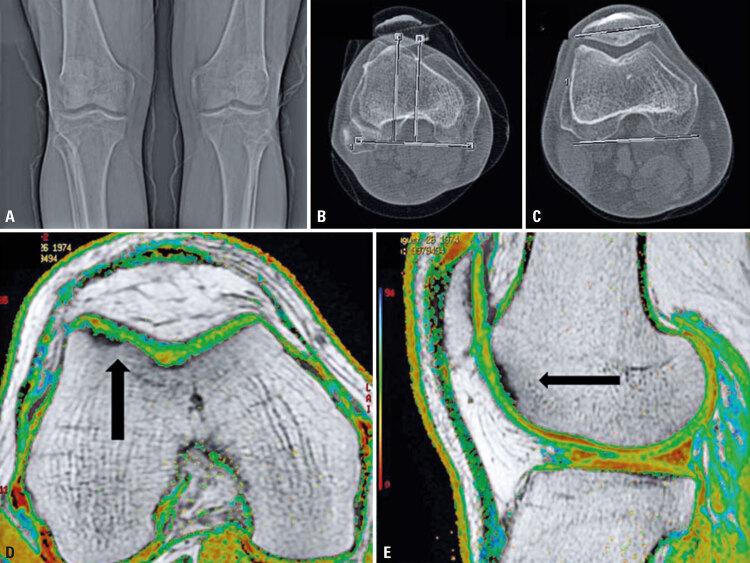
Right knee images before surgery. (A) X-ray with no sign of osteoarthritis; (B) Computed tomography scan with normal TT-TG index (14mm) and C) patellar tilt (7 degrees); (D) T2-weighted magnetic resonance image in the axial plane with focal chondral lesion in the trochlear region (arrow); (E) T2-weighted magnetic resonance image in the sagittal plane view showing the same lesion (arrow)
